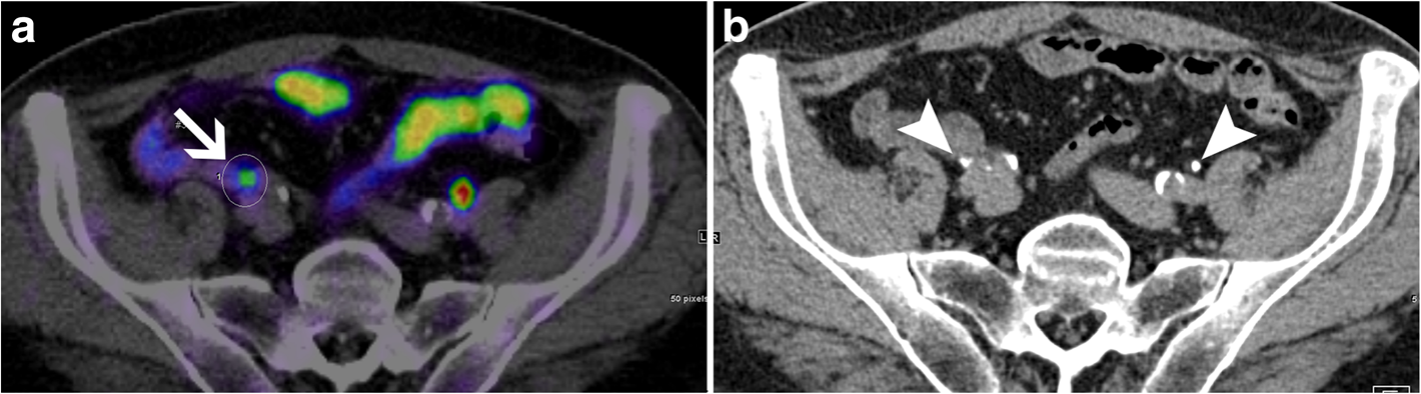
Increase of diagnostic confidence by integrating CTU into ^68^Ga-PSMA-11 PET/CT with CTU. a shows a focal tracer accumulation in the right iliac retroperitoneum (arrow in a) with anatomical assignment to the right ureter with confidence score 1. Anatomical assignment is hampered by proximity of the focus to small intestine and iliac crossing of the right ureter. Using ^68^Ga-PSMA-11 PET/CT with CTU and delineation of both ureters (arrow heads in b), the focus was assigned to right ureteric excretion with confidence score 3
